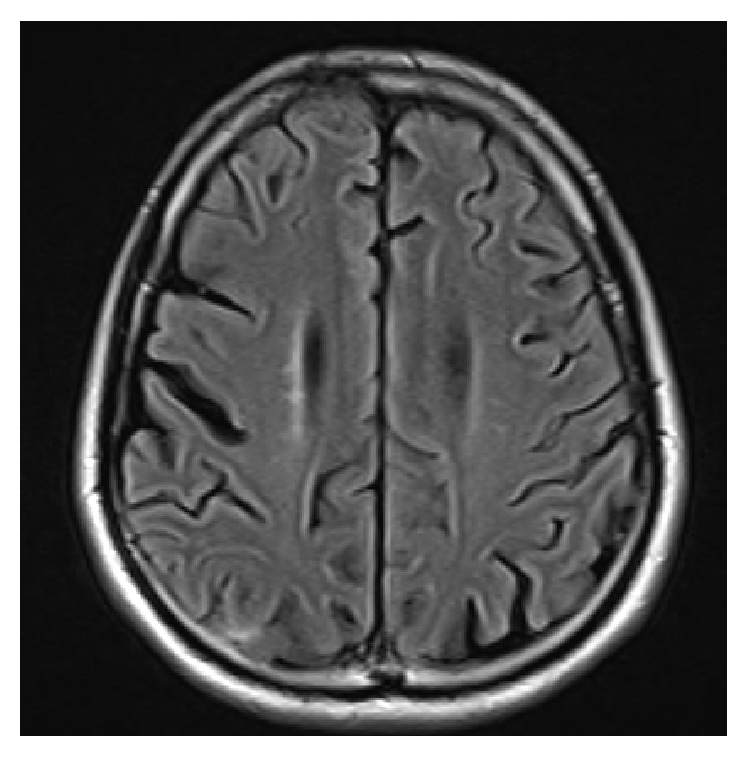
Brain MRI finding 2 years after treatment. T2 FLAIR image (1.5 T; TR 10000 ms, TE 108 ms). MRI performed 2 years after treatment no longer shows linear hyperintensity of the leptomeninges.
